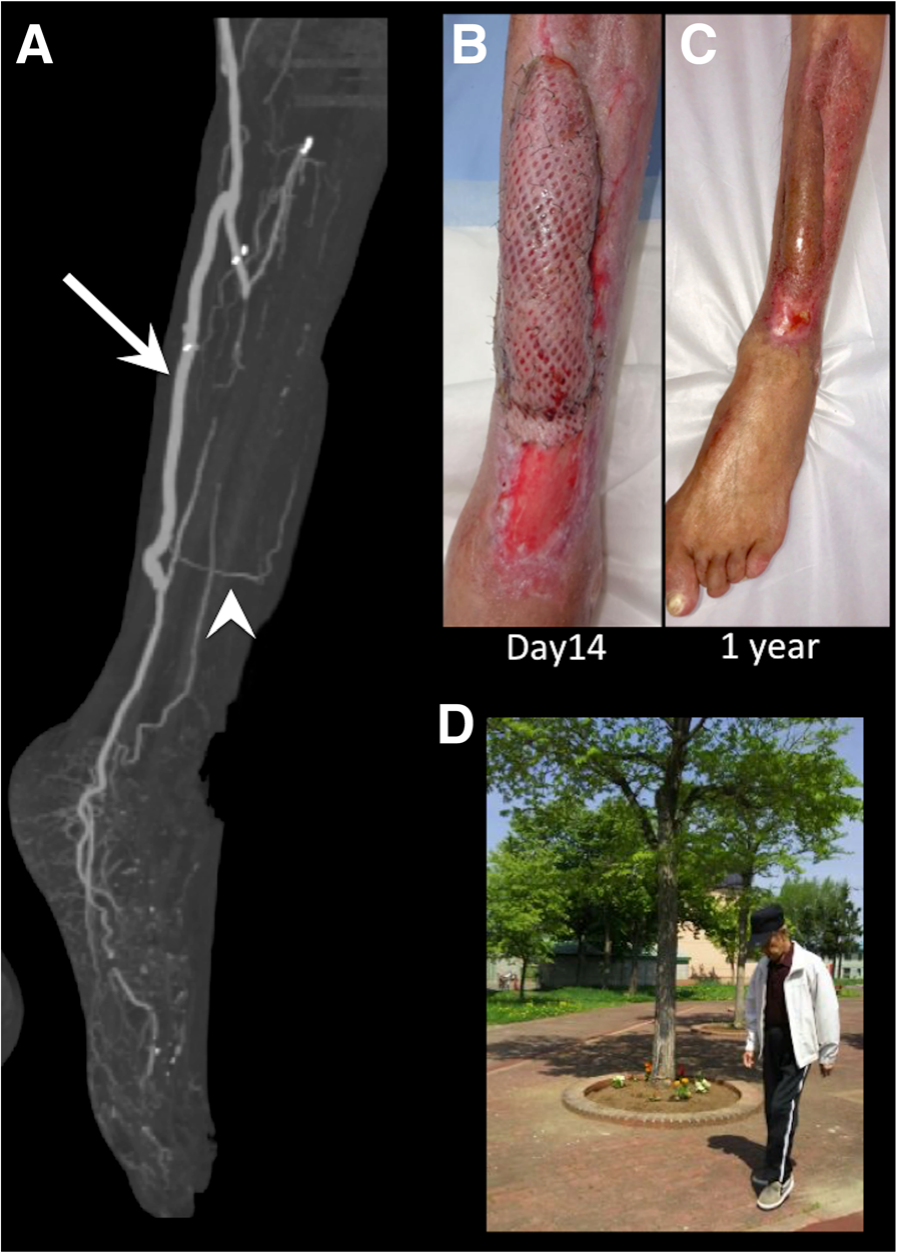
a Computed tomography after the second surgery showed the patent jump bypass to the distal posterior tibial artery (arrow) and the pedicle of the free gracilis muscle flap (arrowhead). b Postoperative photographs showing the gracilis muscle flap covered with a meshed split-thickness skin graft at postoperative day 14. c Photograph 1 year after muscle flap coverage. d Photograph 1 year after operation showing the functional limb salvage with ambulatory status
